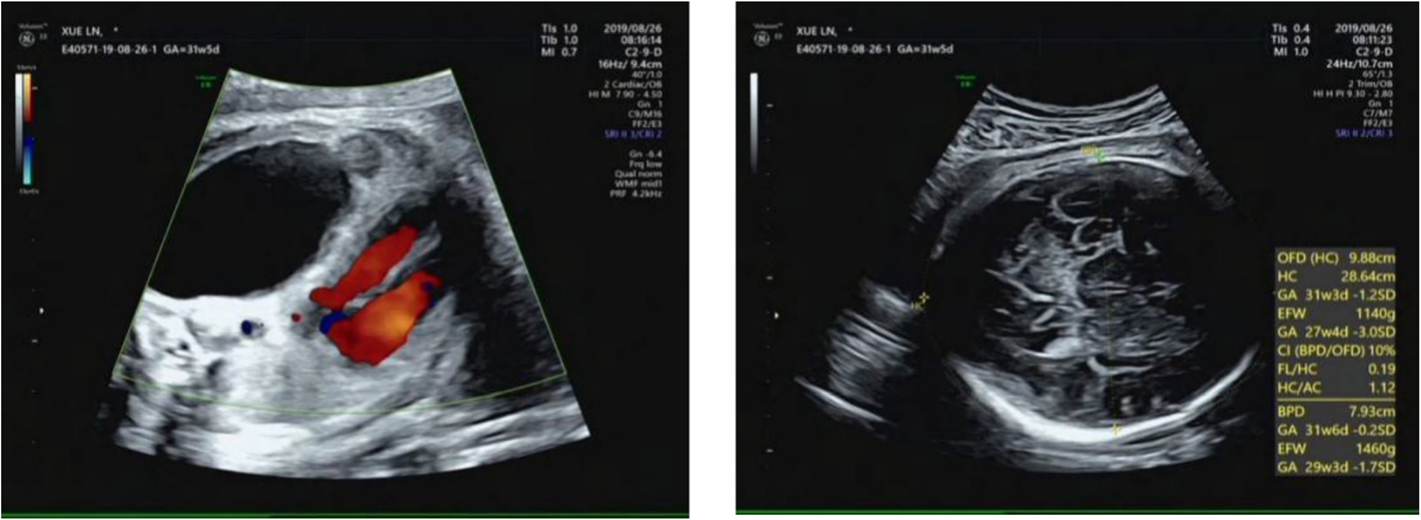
Antenatal ultrasound scan at 30 + 2 weeks gestation shows an echo-free cyst (5.4 cm × 3.3 cm × 5.6 cm) with a blood flow signal from the pulmonary circulation, a head circumference of 28.6 cm, and a CVR of 1.82
